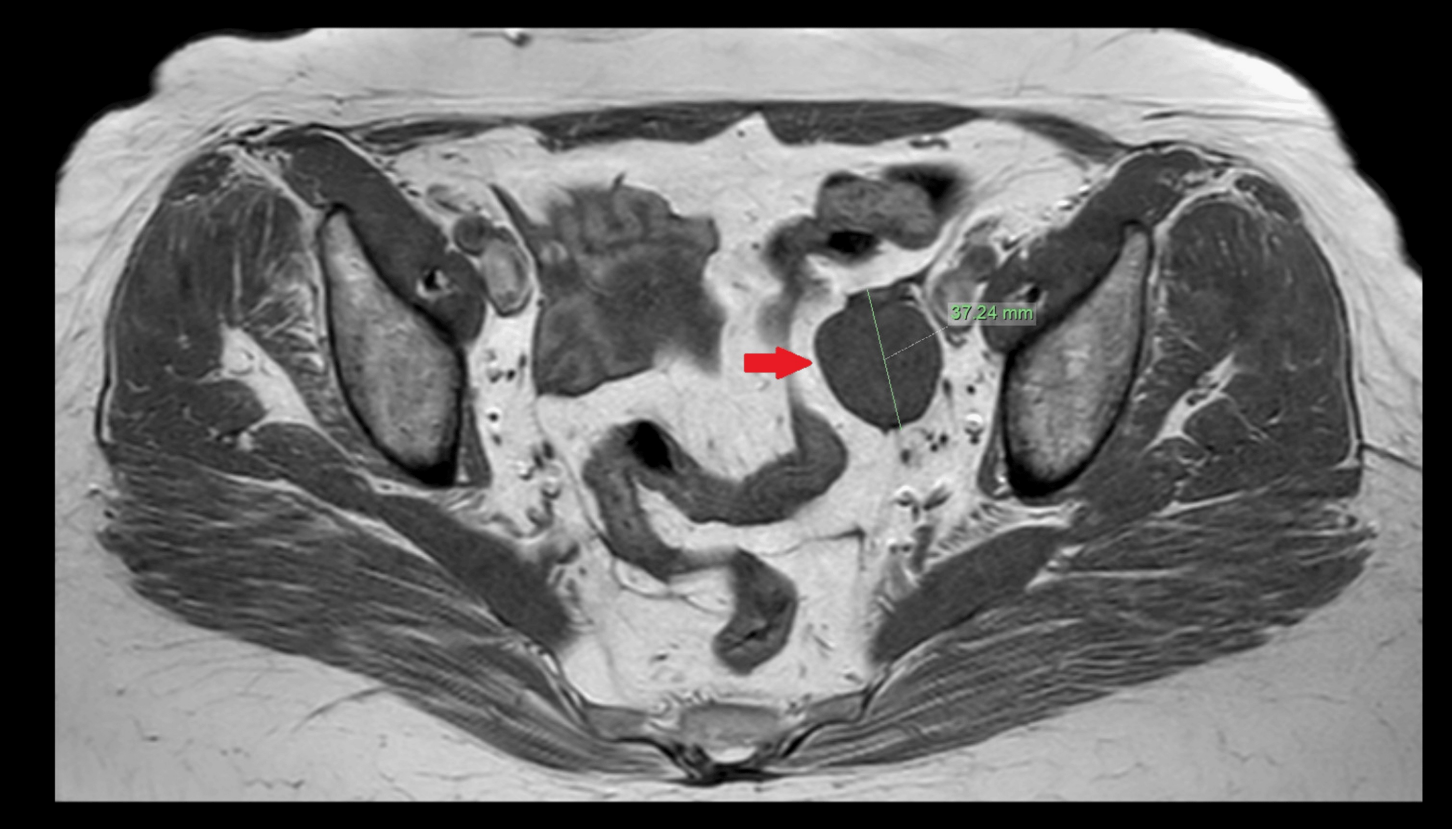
The red arrow highlights a 4-cm-diameter left adnexal mass with low signal on MRI. MRI, magnetic resonance imaging
